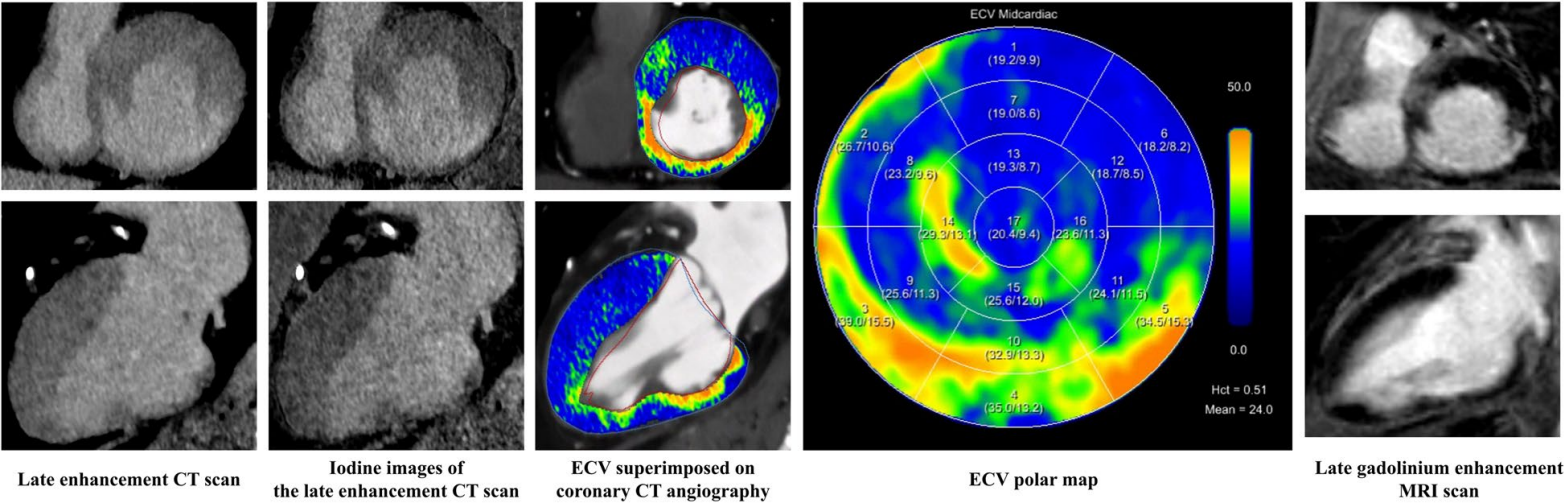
Late enhancement scan and extracellular volume (ECV) calculation in an 89-year-old male patient. Conventional images and iodine maps from the late enhancement scan show increased transmural contrast enhancement of the inferoseptal, inferior and inferobasal myocardium. Elevated ECV_CT_ values were observed in these regions suggesting a focal scar. Findings were confirmed by cardiac MRI. Note the identical extent of the scar on both modalities
